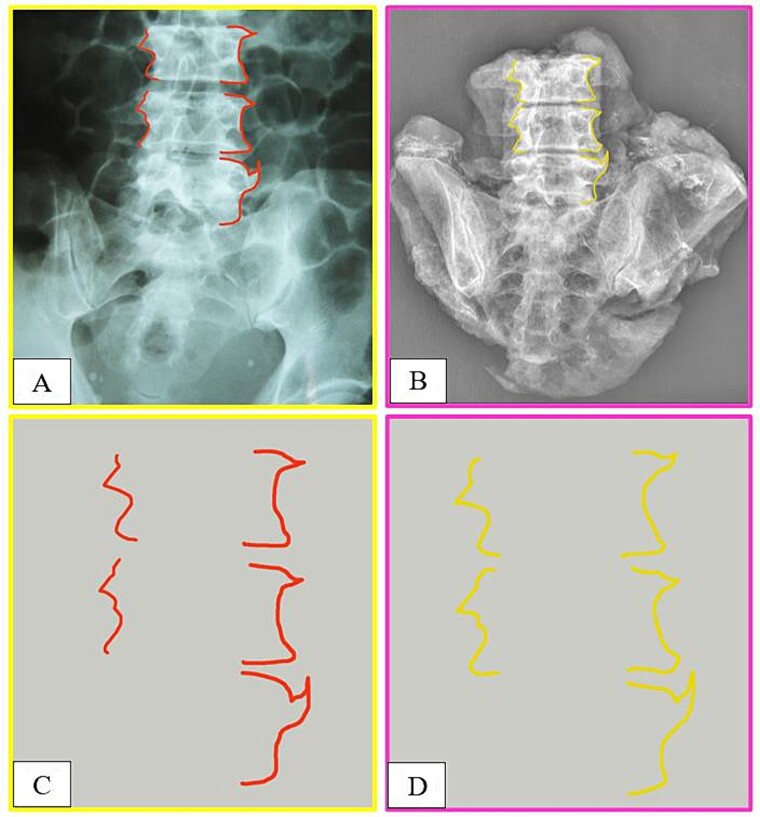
Intravitam (A) and postmortem (B) X-ray confrontation of the lumbar spine. Comparison of the antemortem (C) and postmortem (D) templates.
